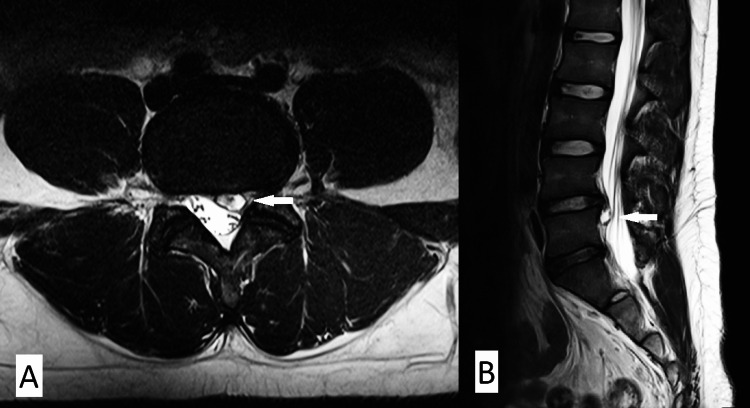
Lumbosacral MRI. Axial (A) and sagittal (B) views demonstrating discal cyst (white arrow)
